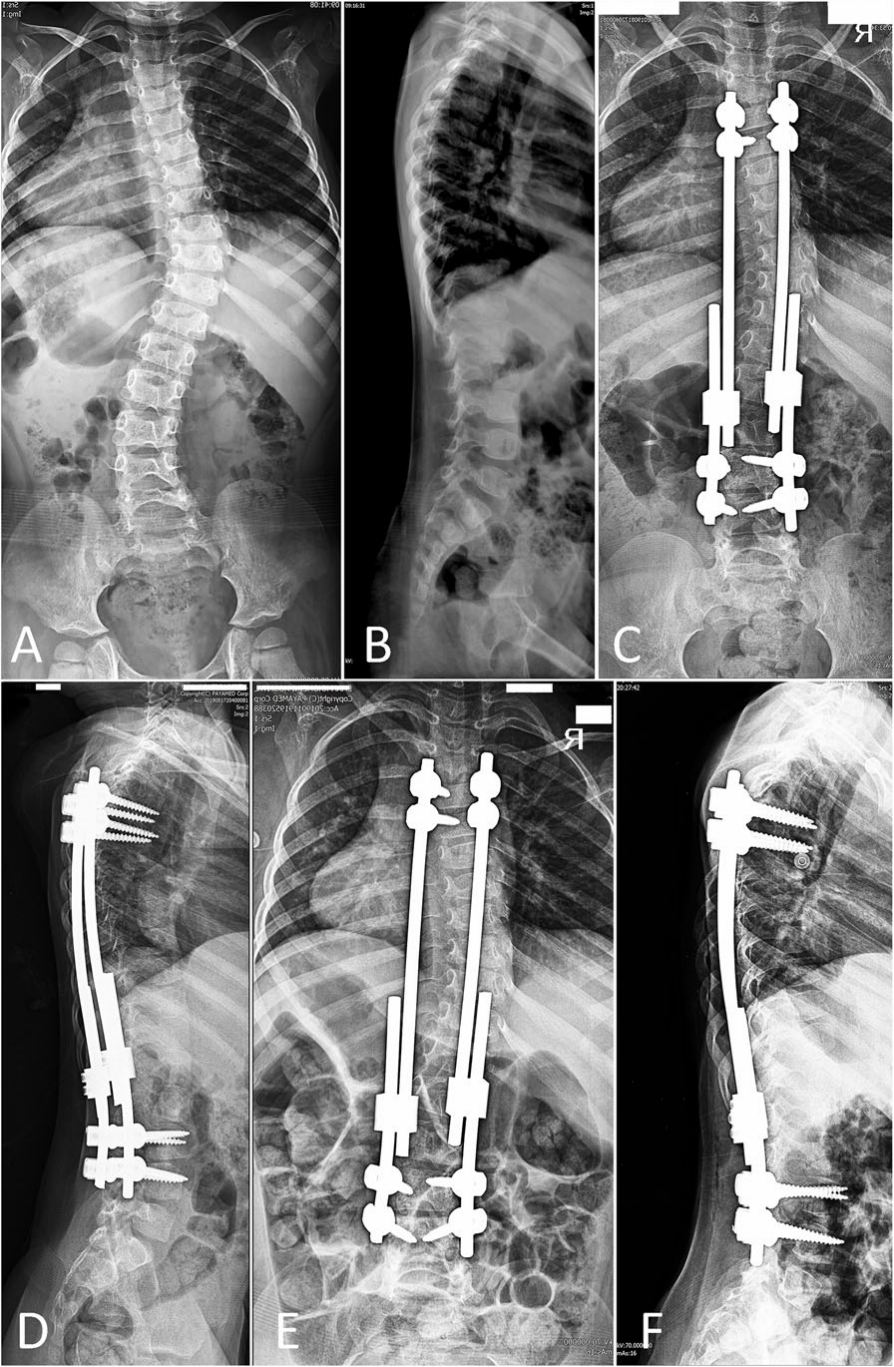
A five-year-old girl with idiopathic early-onset scoliosis (a-b) underwent dual growing rod instrumentation with all-pedicle screws (c-d). Latest follow-up radiographs (e-f) following four rounds of surgical lengthening reveals excellent correction with a proximal junctional angle of 5°. The patient is extremely active and happy with the results
